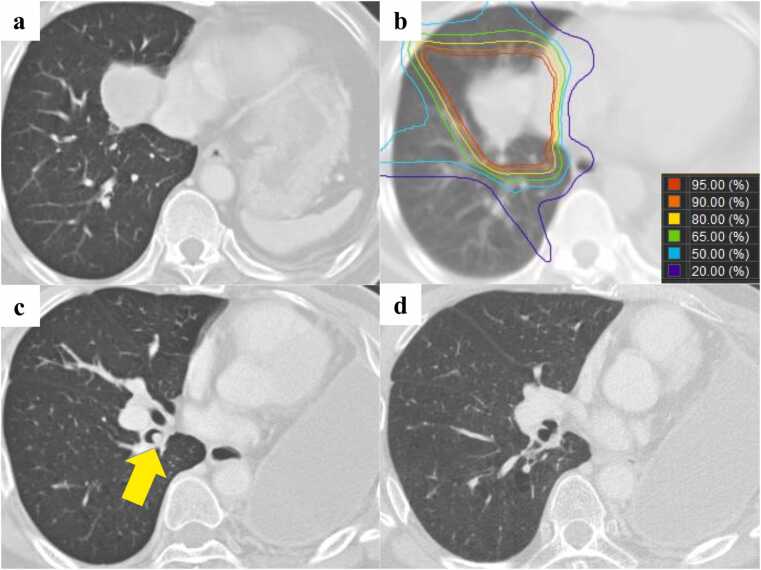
Computed tomography images of case 1 at the time of the first proton beam therapy (PBT) and at recurrence after PBT. a) The primary tumor invading the mediastinum in the right S7 before the initial PBT. b) The dose distribution of PBT. c) Recurrence in the mucosa of the right B7 at 7 months after PBT (arrow). d) The right B7 after bronchoscopic electrocautery for the recurrence.
